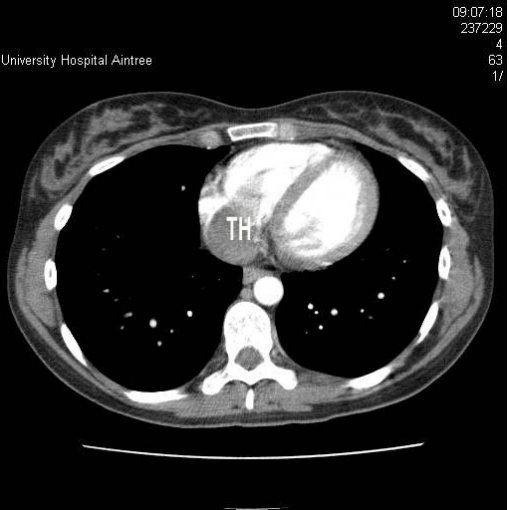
Computed tomography scan of the chest showing the tumour thrombus (TH) in the right atrium.
